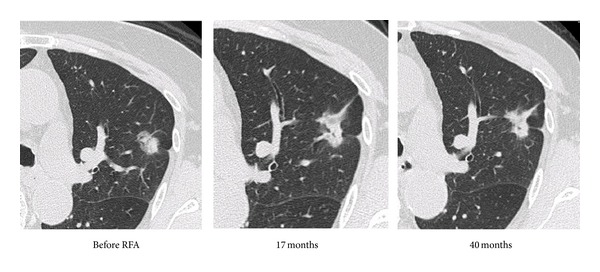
Serial CT findings before and after bronchoscopy-guided cooled radiofrequency ablation (RFA) in case 2.
